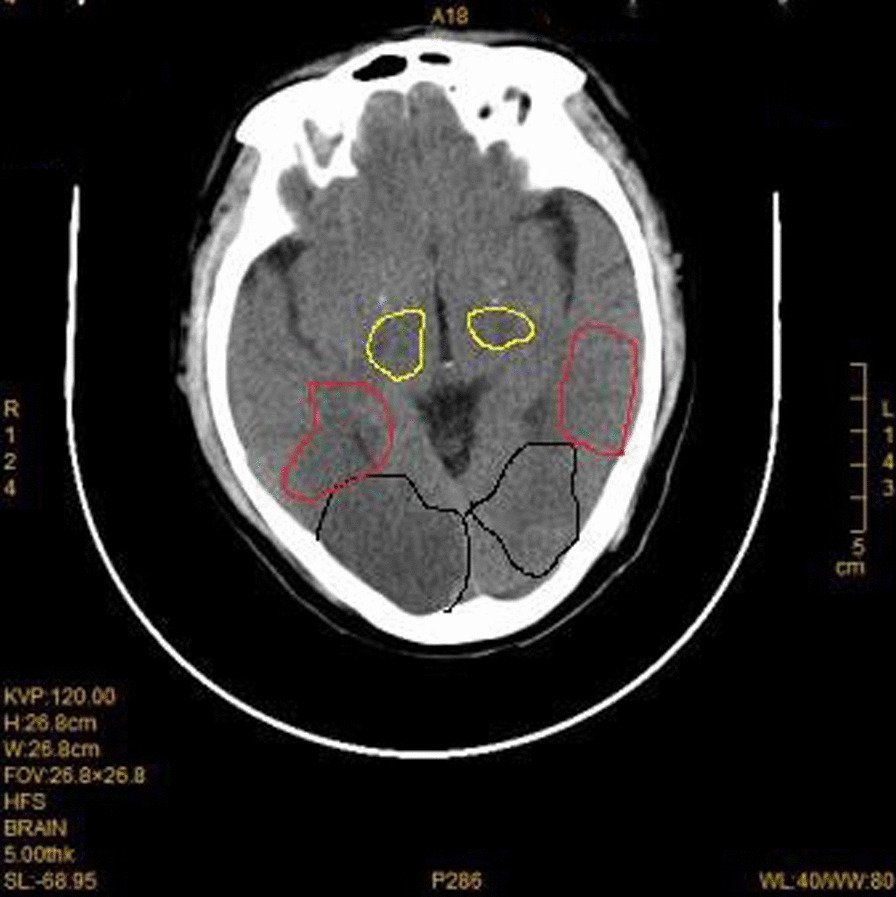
Three days after air embolism, CT showed a patchy low-density shadow in bilateral thalamic basal ganglia (yellow area), temporal lobe (red area) and occipital lobe (black area), which was the cerebral infarction lesion after air embolism
